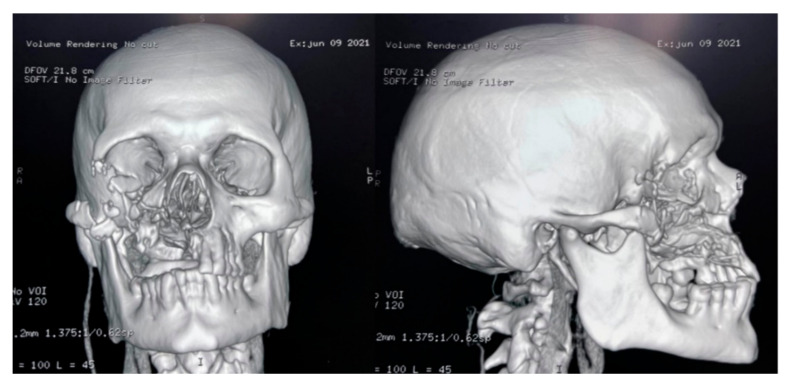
CT-scan of the head after the surgery.
